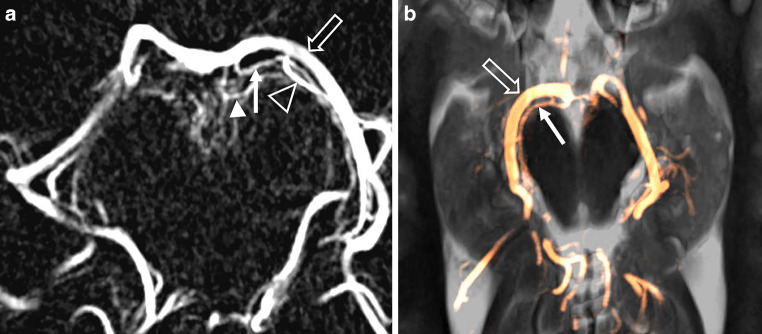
a 3D rotational digital subtraction angiography, axial maximum intensity projection (MIP) of the posterior cerebral artery (PCA, open arrow) with collicular artery (CA, arrow), accessory CA (arrowhead) and posterior medial choroidal artery (PMCA, open arrowhead). b axial MIP of the PCA (open arrow) and the CA (arrow) projected onto axial T2 weighted MR images
